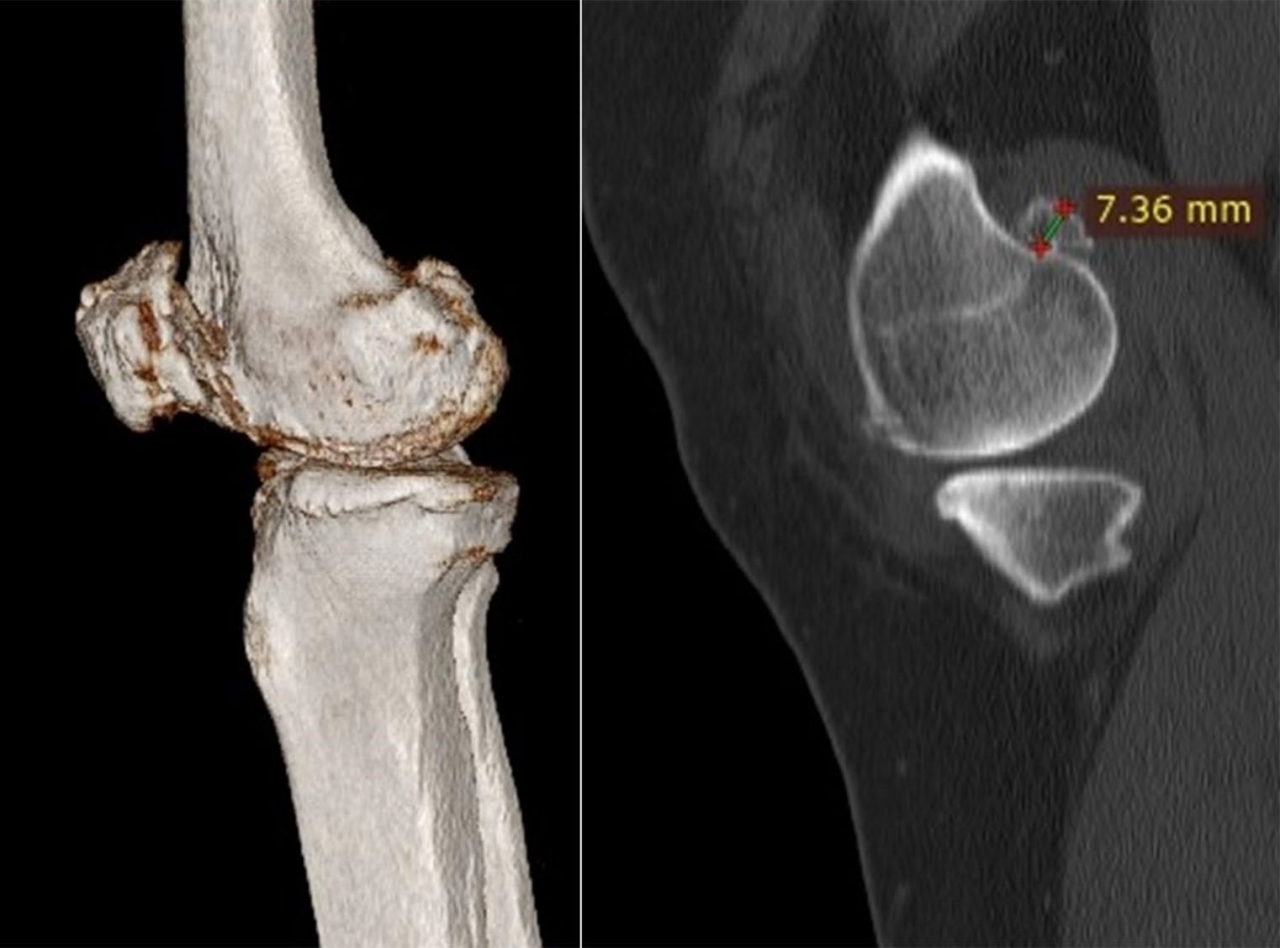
CT scans for preoperative evaluation of the posterior condylar osteophyte position
